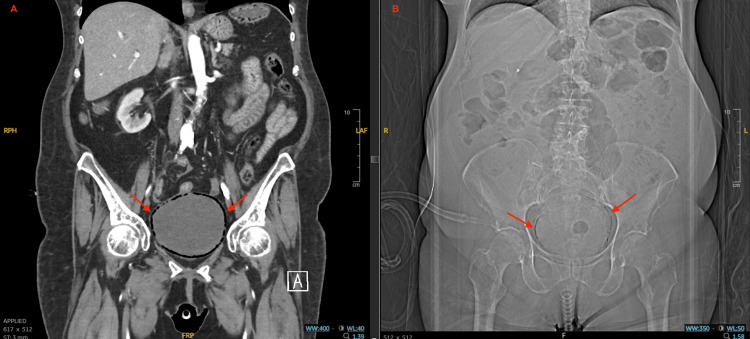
Abdominal coronal CT angiography and abdominal X-ray – emphysematous cystitis. (A) Coronal CT angiography of the abdomen and pelvis showing intramural bladder wall gas (red arrows) consistent with emphysematous cystitis. (B) Abdominal X-ray demonstrating mottled radiolucencies overlying the bladder (red arrows), corresponding to gas within the bladder wall. While X-ray can detect intramural air, its sensitivity and anatomical detail are inferior to CT.
